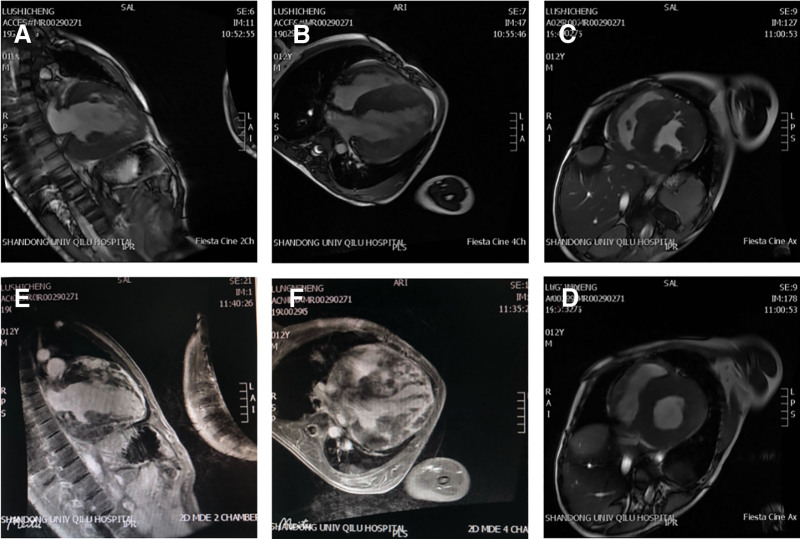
Cardiac magnetic resonance imaging (CMR) of the patient. (A, B) Diffuse thickening of the left and right ventricular myocardium; (C, D) MR first-pass myocardial perfusion imaging showing multiple areas of hypoperfusion in the left ventricular myocardium, suggestive of myocardial ischemia; (E, F) MR delayed-enhancement scanning revealing multiple foci of abnormal enhancement in the left and right ventricular myocardium, indicative of scarring or fibrosis.
